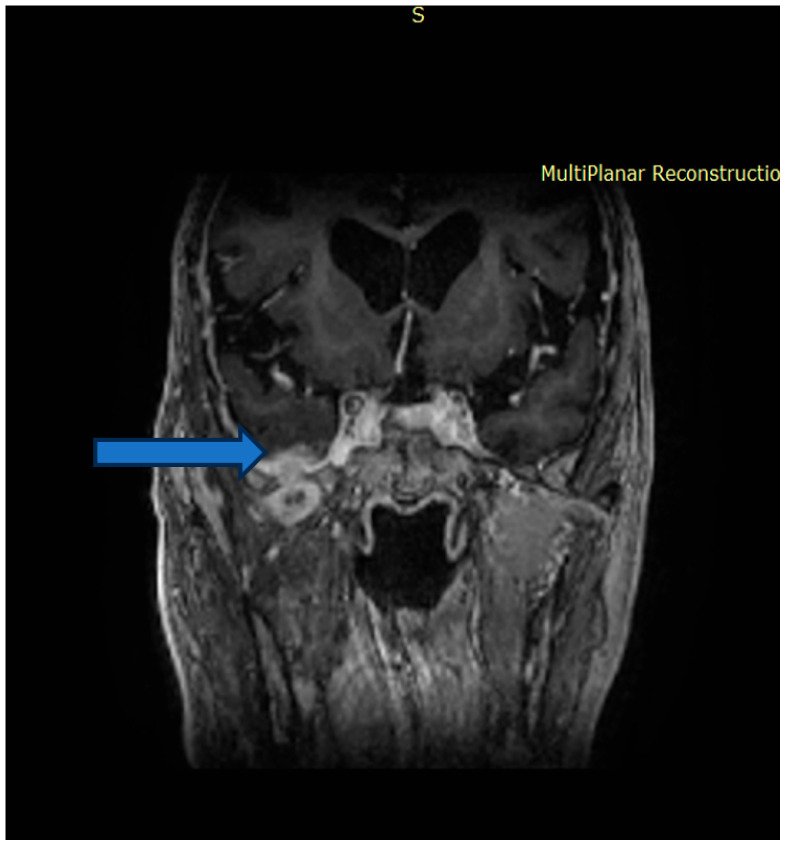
Magnetic resonance imaging before surgery. Invasion of the tumor in the dura mater (marked with arrow).
